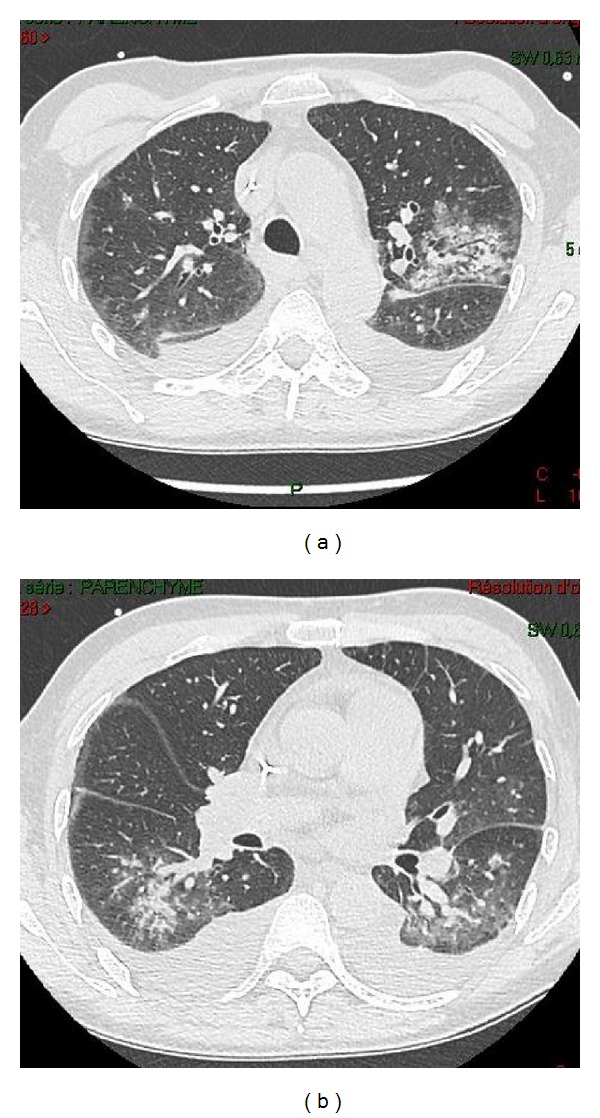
Thoracic computed tomography scan of the patient, showing bilateral and diffuse extension of the infection. (a) Bilateral subpleural and peribronchial infiltrates with bilateral pleural effusion in the superior lobes. (b) Disseminated alveolointerstitial infiltrates in the basal areas.
